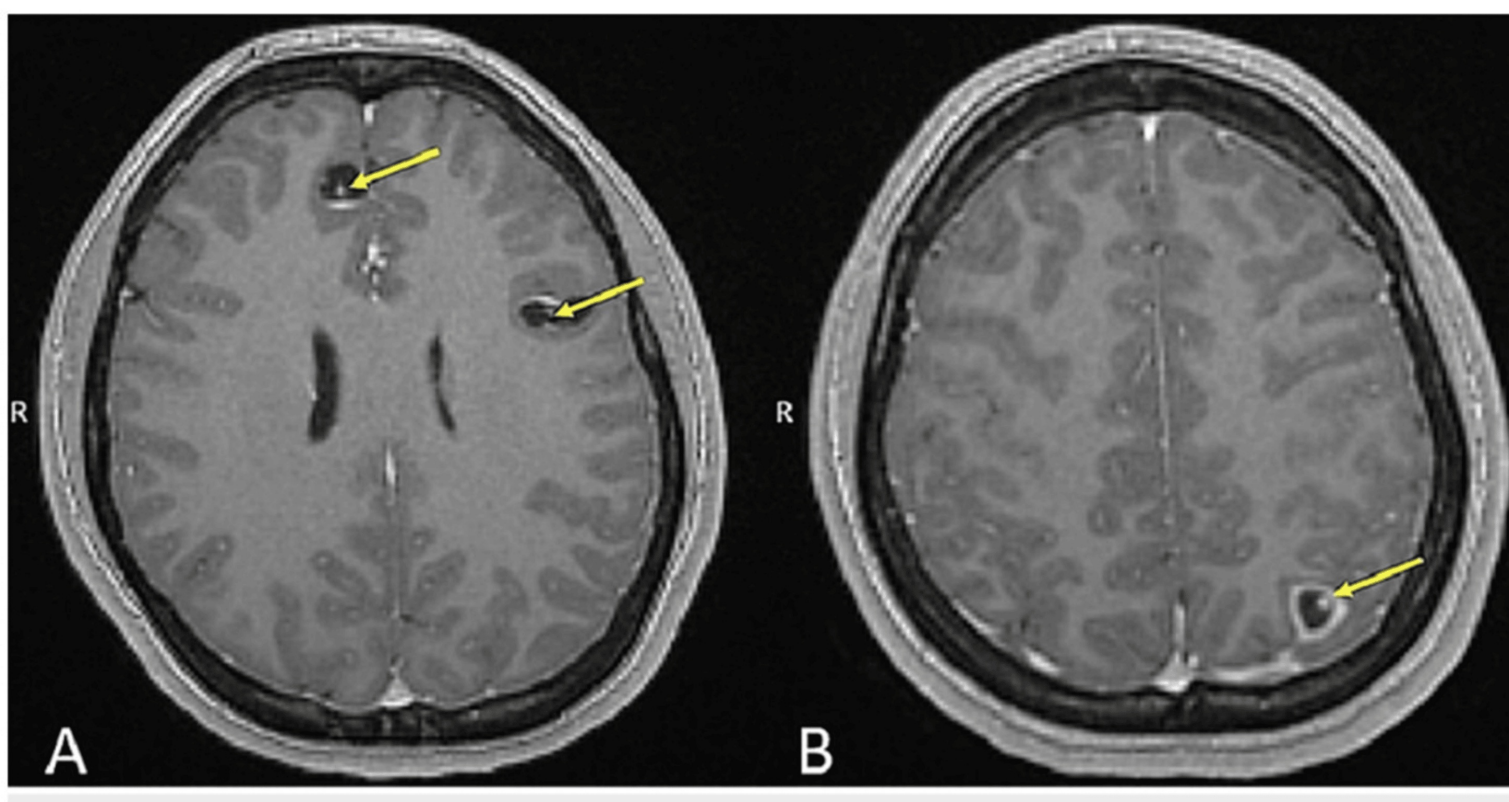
Scolex within the cysts seen on MRI Permission to use image granted by Dr. Norman Beatty [8]
